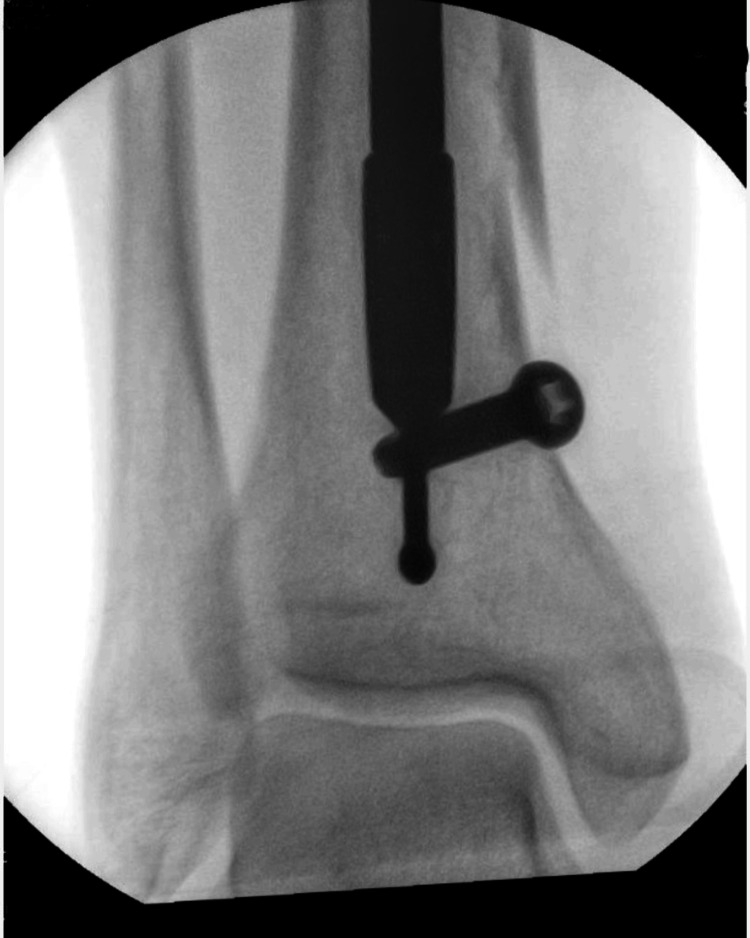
An X-ray showing the nail obstructed by the poller screw.
